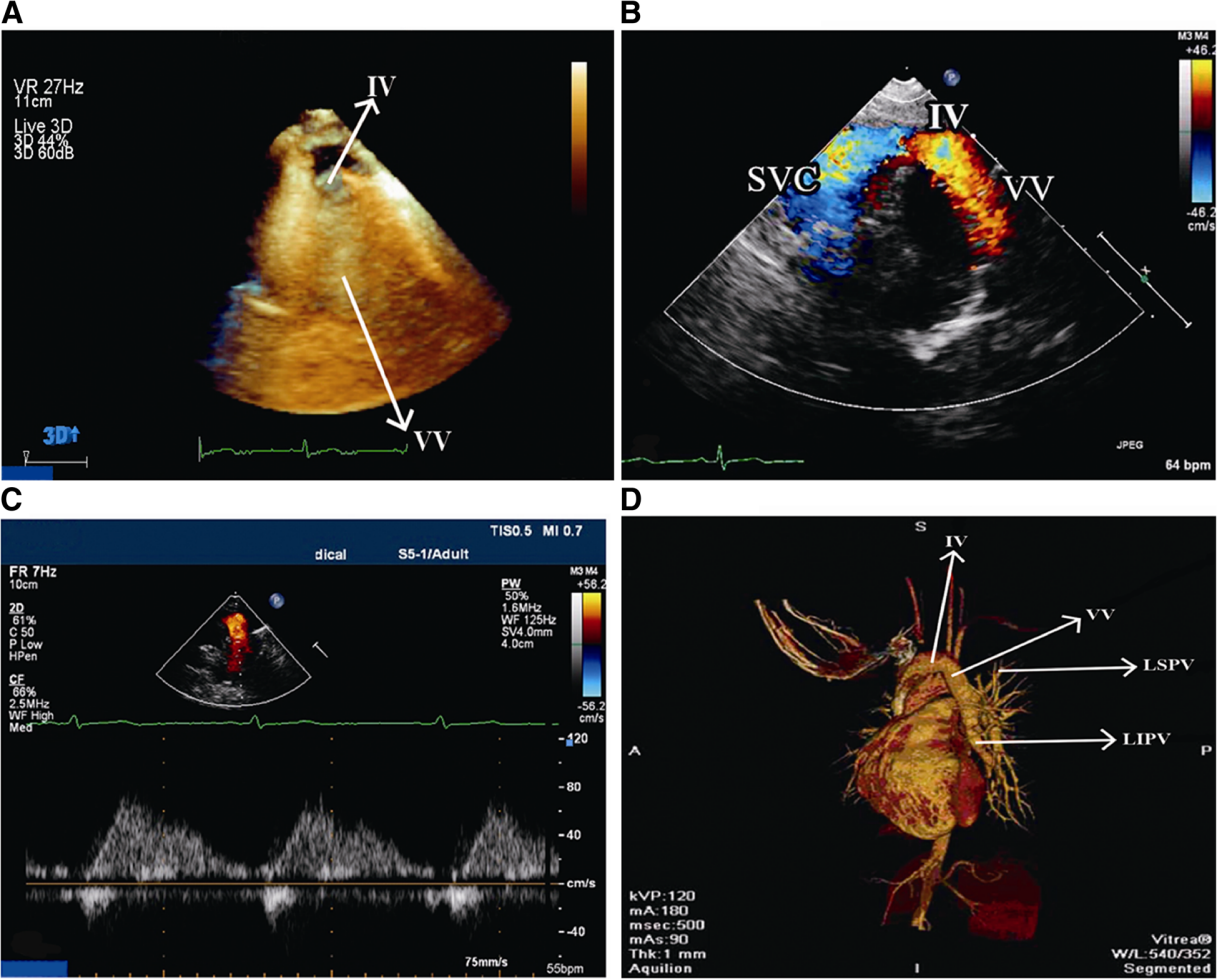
Four images for detecting partial anomalous pulmonary venous connection (PAPVC). (A) Three dimensional transthoracic echocardiography showed PAPVC. (B) The suprasternal short-axis view with color Doppler revealed left pulmonary veins connecting with the innominate vein via a vertical vein. (C) Upward blood flow in the vertical vein was confirmed by pulsed Doppler imaging. (D) Multidetector computed tomography angiography was performed to confirm the structure of the anomalous venous system. IV: innominate vein; SVC: superior vena cava; VV: vertical vein.
